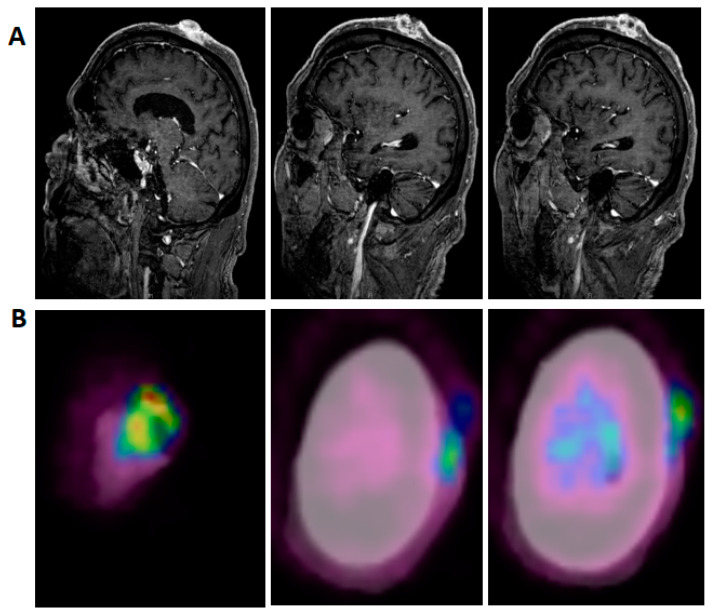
Representative pre-treatment, post-contrast, T1-weighted, sagittal MRI images (A) and fused PET-CT images (B) show disease extension predominantly from the vertex scalp to the left lateral temple region.
